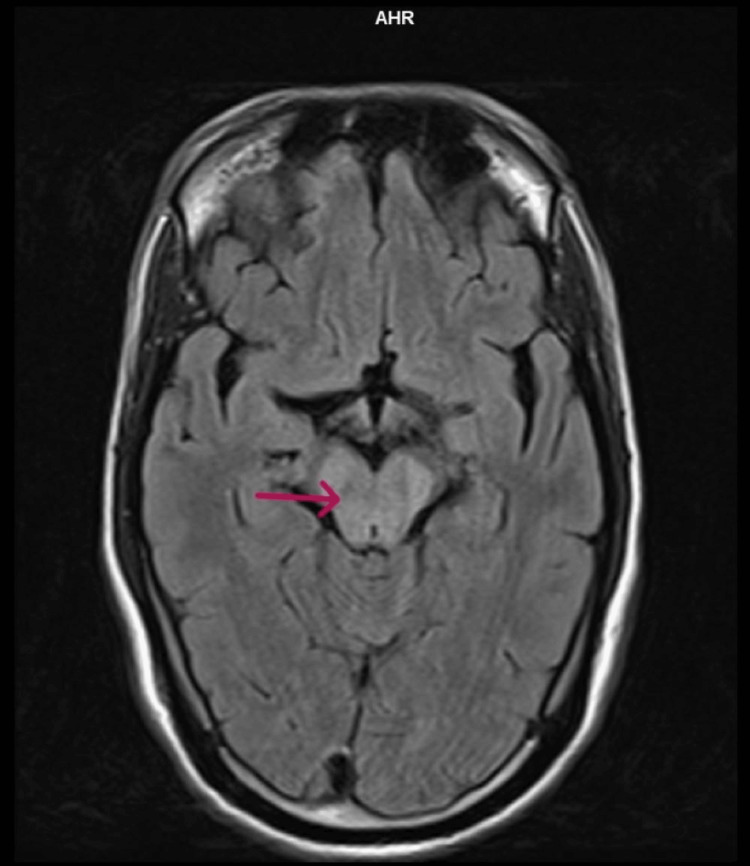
T2 FLAIR hyperintensities involving midbrain (arrow) on the axial section FLAIR: fluid-attenuated inversion recovery
